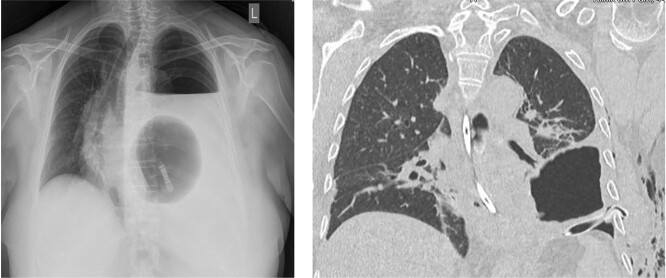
Chest X-ray showing a large left-sided tension seropneumothorax with a right-sided mediastinal shift. Chest CT-scan after insertion of a chest tube.
